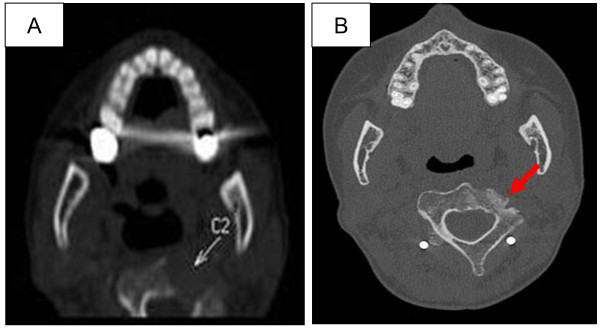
Computed tomography scan of the neck. (A) revealing C2 osteolysis as indicated by the arrow. (B) showing osteocondensation (as indicated by the arrow) in the location of the initial lysis of the C2 vertebrae after 2 months of treatment with erlotinib.
